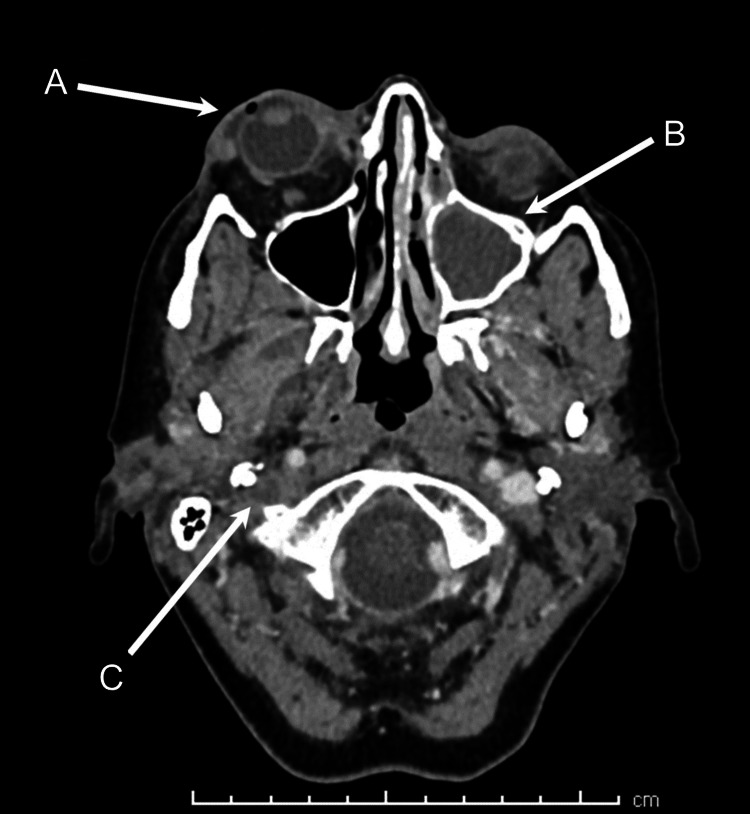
CT image showing right preseptal thickening with an associated gas pocket (A), further opacification of the left maxillary sinus (B), and extension of the thrombus into the right internal jugular vein (C)
